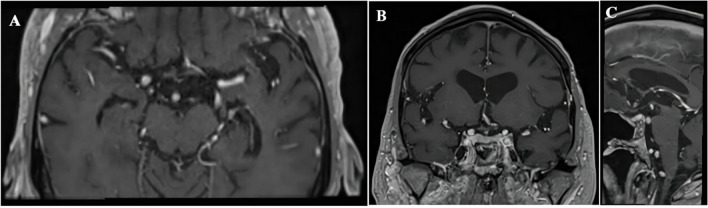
Postoperative T1 weighted MRI with contrast done 6 weeks post resection, shows a gross total resection of the tumor with preservation of the pituitary stalk and optic chiasma
